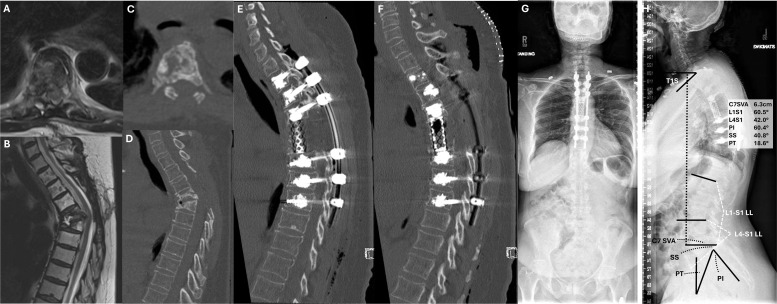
Case 2. Patient with previous T3-T5 laminectomy who subsequently presented to clinic with signs and symptoms of lower extrmity myelopathy. A,B) MR and C,D) demonstrated focal kyphosis at the T5-6 levels with >50% vertebral body collapse and draping or bowstringing of the spinal cord over the kyphotic bodies. E, F) The patient underwent T5-6 corpectomy with titanium mesh cage reconstruction and long-segment instrumented fusion from T2 to T9. G, H) Postoperative upright radiographic films showed elimination of the kyphotic defect with good overall sagittal and coronal balance.
